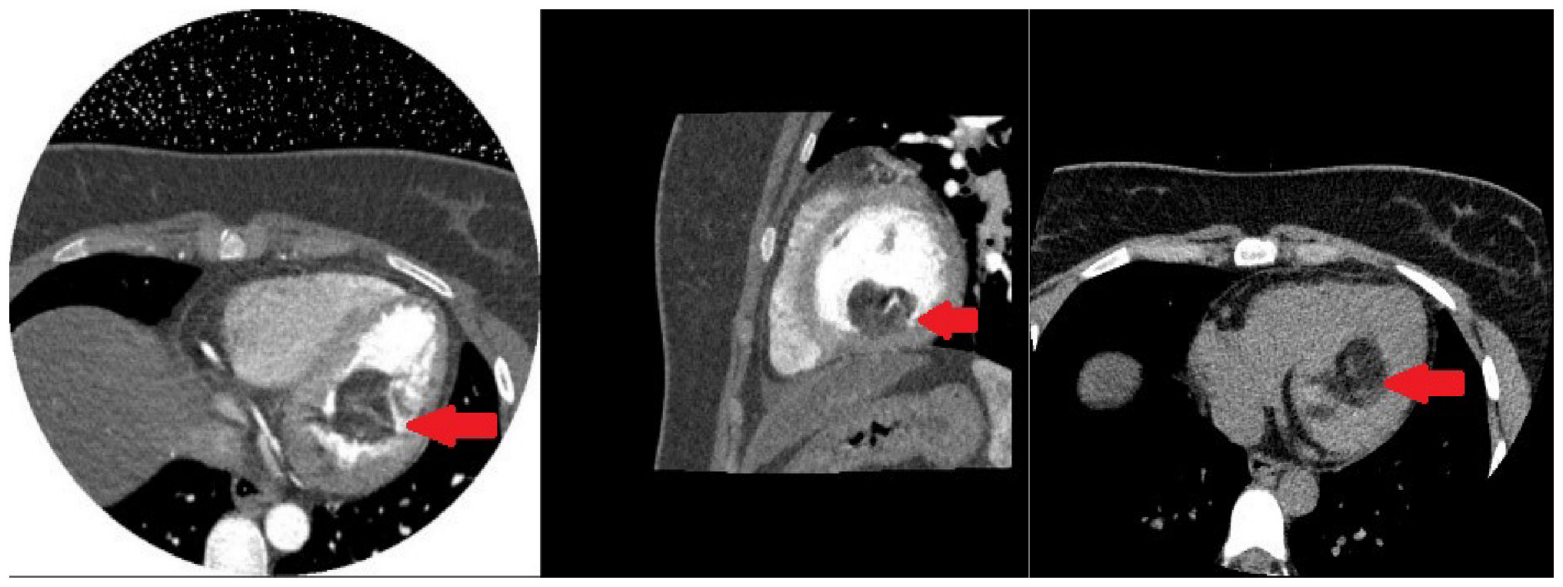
Computed tomography angiography results. The arrow indicates a tumor in the left ventricle, with a size that is partially adherent to the ventricular wall.
